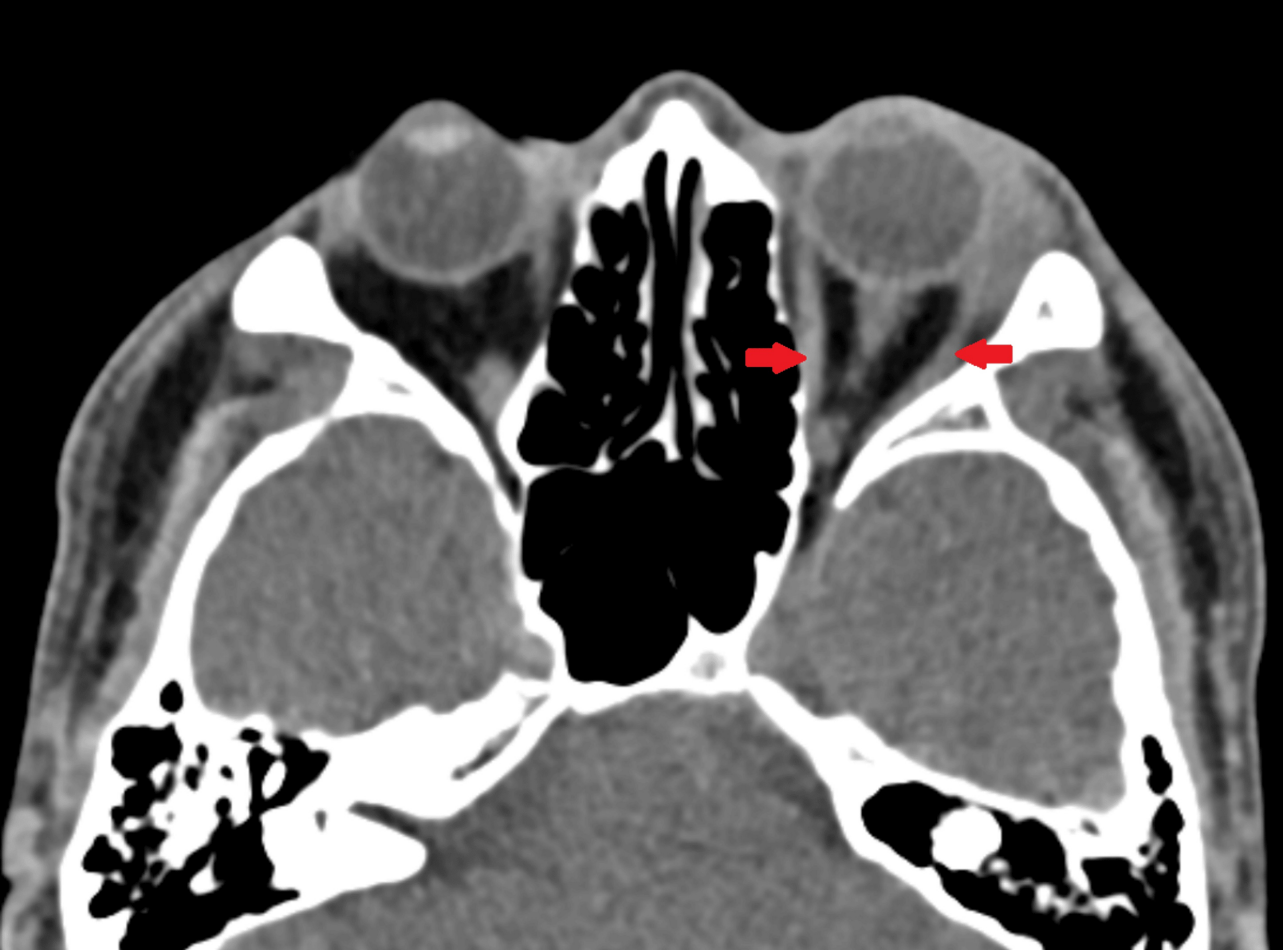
CT orbit showing no tendonitis or myositis (arrows).
